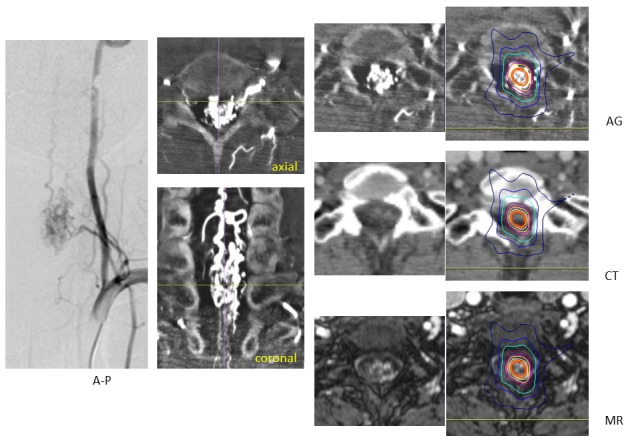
A spinal arteriovenous malformation. Digital subtraction spinal angiography showing an intramedullary AVM in the cervical spinal cord (left). The 3-D reconstruction from the axial images of rotation angiography (middle line) showing the nidus and draining veins. The dose plan targeting the nidus while sparing the surrounding spinal cord, with the steepness of the isodose line on axial images of an angiogram (AG) fused to CT and enhanced MR images (right line).
